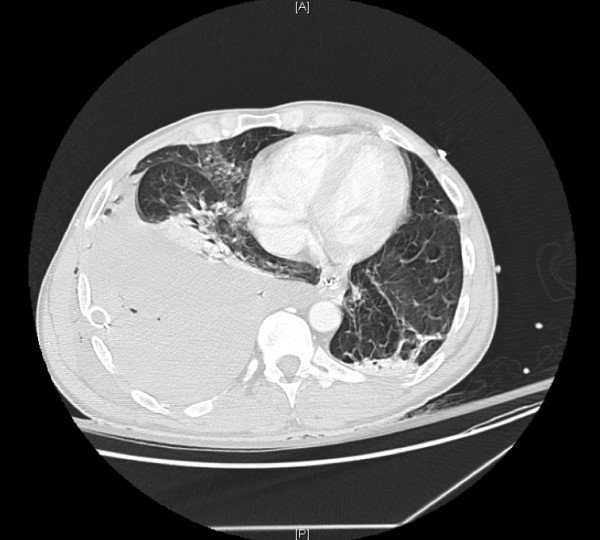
CTA of chest revealing large residual clot in the right hemi-thorax. This study was performed in an attempt to localize the bleeding source in our patient. The study was negative in terms of identifying an anatomic source of bleeding (most relevant with respect to examination of the great vessels in the thoracic outlet, albeit falsely negative). However, this study served as a proxy for the post-thoracostomy chest x-ray and identified the insufficient drainage of the right chest with the thorocostomy tube in place.
